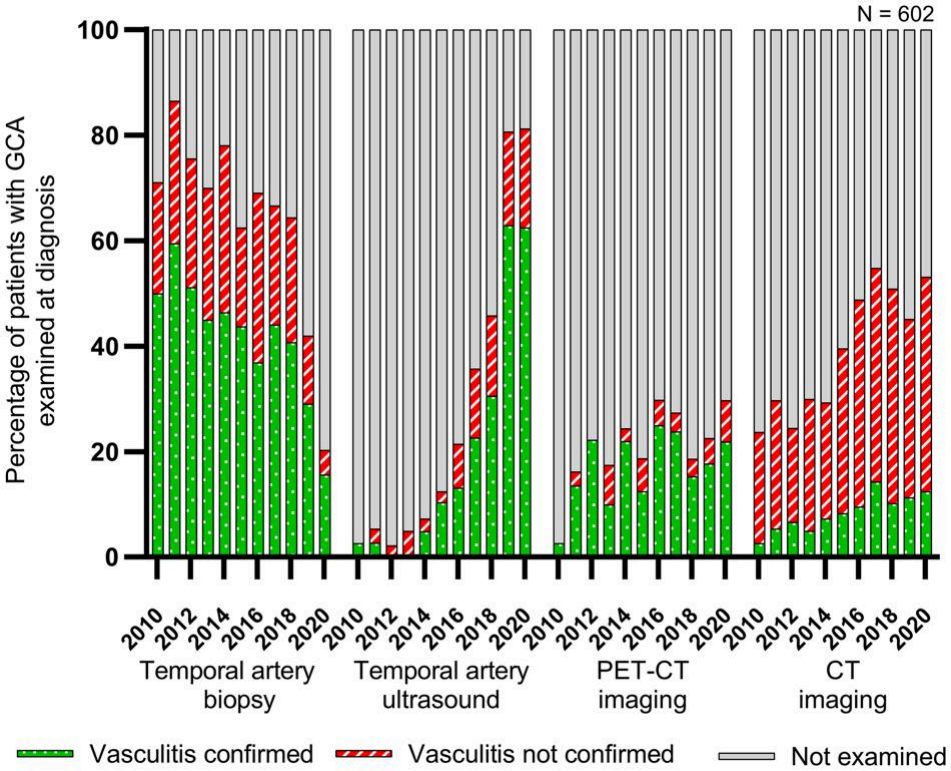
The annual (from 2010 to 2020) percentages of different investigation methods performed at diagnosis for patients with GCA. PET-CT means ^18^F-fluorodeoxyglucose PET-CT. CT means any CT imaging of arteries
